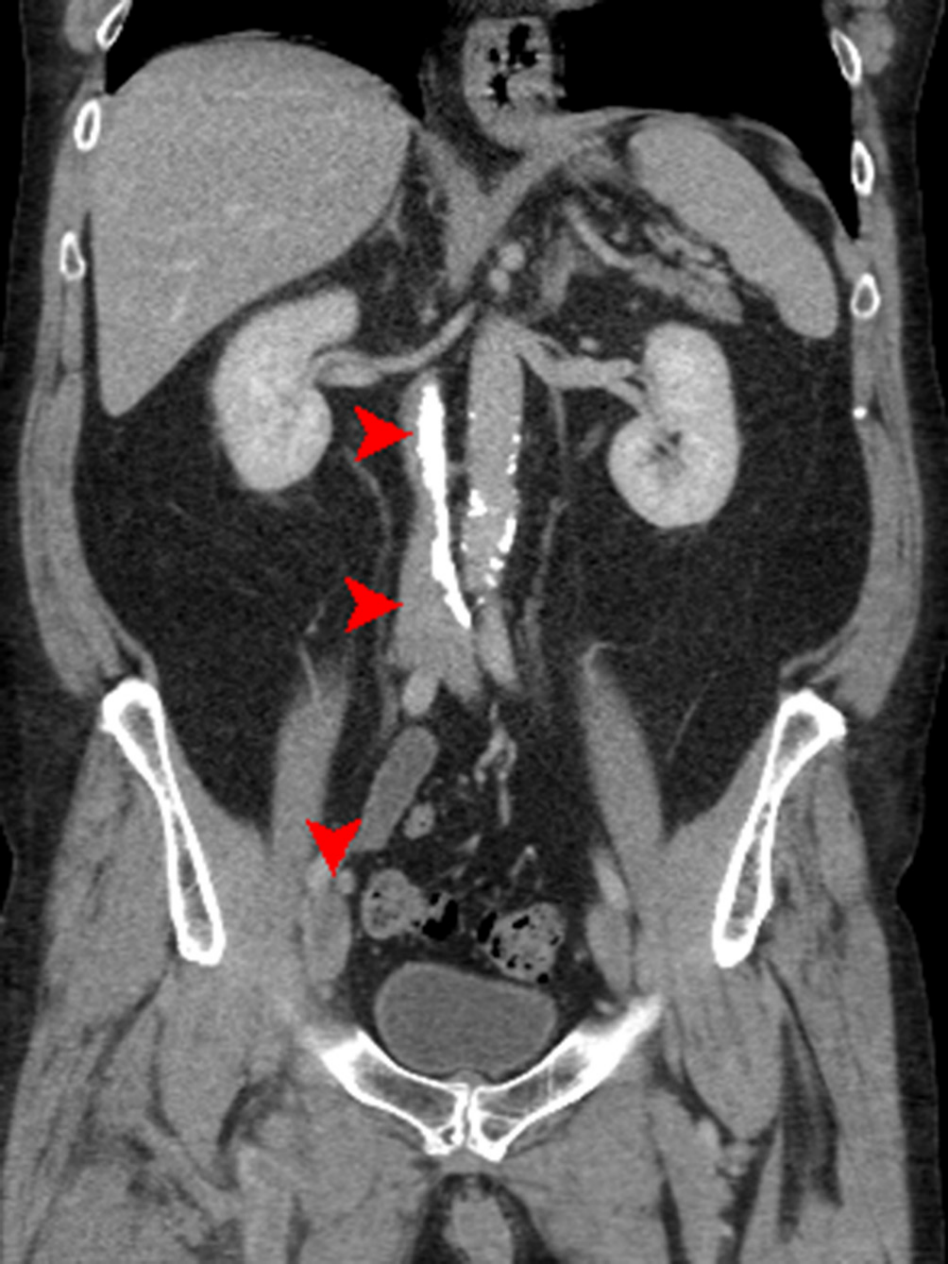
Coronal CT showing calcification of the IVC and thrombus in the terminal
